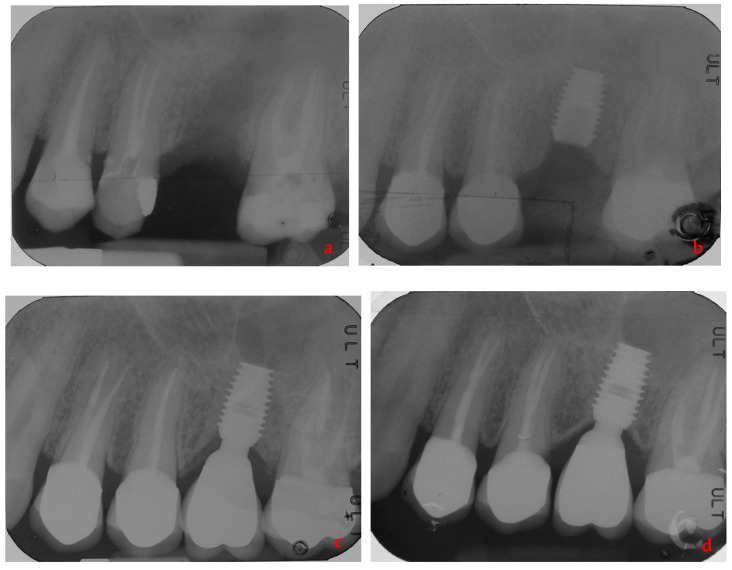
Single implant placed in 2.6 site (4.5 × 8 mm) of a male patient without history of periodontal disease: (a) pre-operative radiograph before implant placement; (b) radiograph obtained at implant placement; (c) radiograph obtained at time of loading; (d) radiograph obtained at 3-year follow-up.
